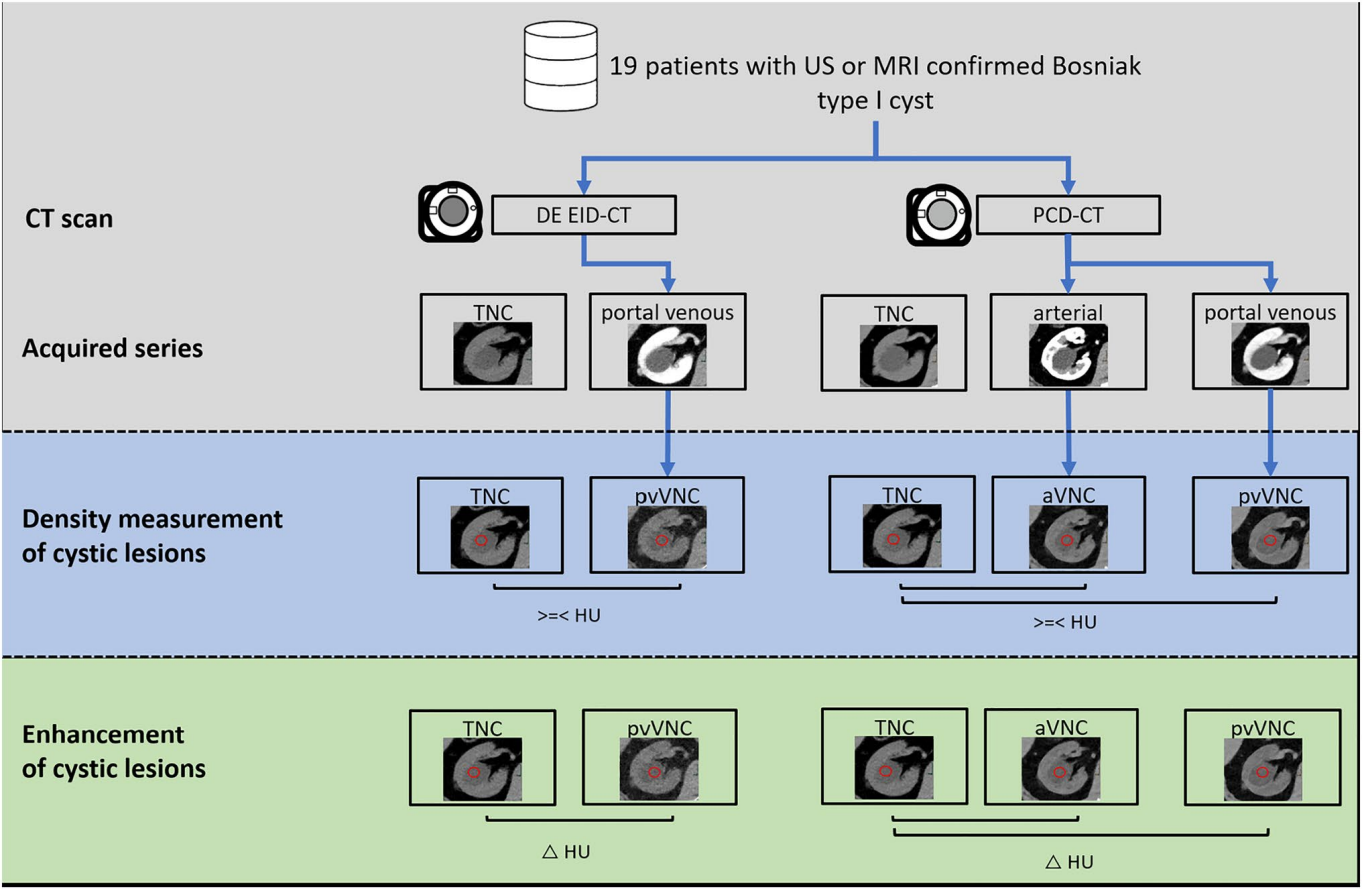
Overview of the study design. All patients received clinically indicated multiphasic abdominal CT in PCD-CT. If a historical multiphasic abdominal CT on DE EID-CT was not already available, the clinically indicated follow-up examination was performed on DE EID-CT. aVNC = arterial virtual non-contrast; pvVNC = portal venous non-contrast; TNC = true non-contrast; PCD-CT = photon-counting detector CT; DE EID-CT = dual-energy integrating detector CT; MRI = Magnetic resonance imaging; US = Ultrasound
